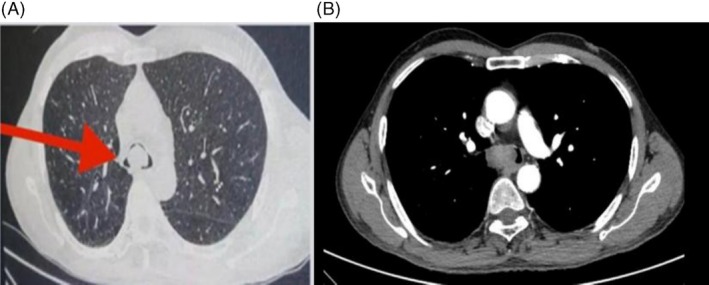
(A) The axial CT section of thorax, Lung window at the level of distal trachea. (B) The axial CT section of thorax, mediastinal window at the level of carina, the post‐contrast image. Both showed a 3 × 2.1 × 1.4 cm well marginated, lobulated, soft tissue density mass with heterogeneous post contrast enhancement at the distal trachea, extending into both proximal main bronchi.
